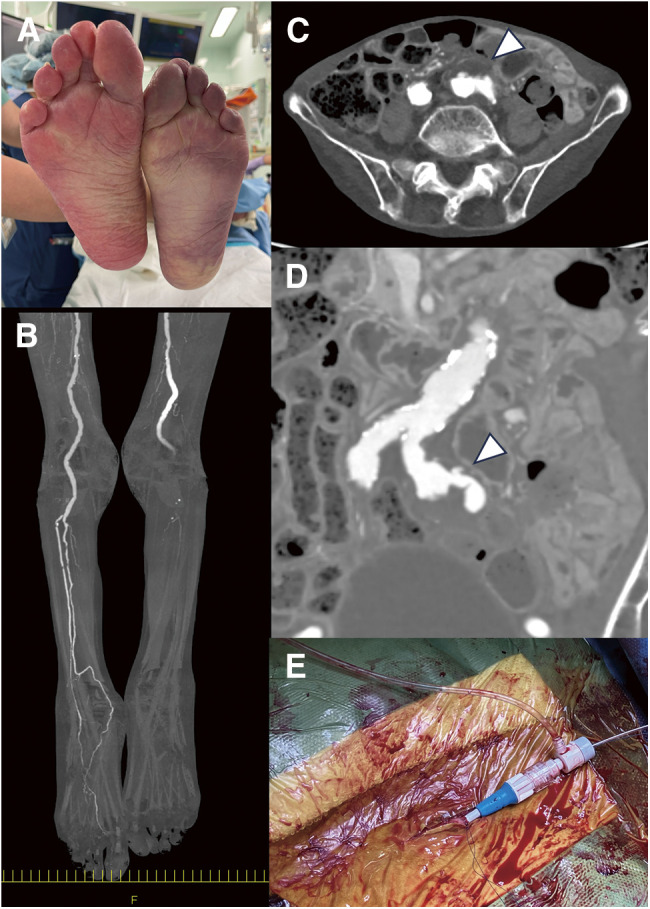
Fig. 1 Cyanotic alteration in the left foot (A). CTA revealed an acute occlusion in the left lower extremity artery, distal to the popliteal artery, and underlying arterial lesions in the right infrapopliteal arteries (B). The left CIAA with a thrombus was identified as the source of the embolism (C and D). The left CFA was exposed and an 8-French sheath was inserted (E). CTA: computed tomography angiography; CFA: common femoral artery; CIAA: common iliac artery aneurysm
